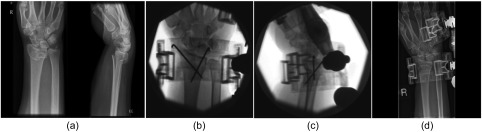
Radiographs and fluoroscopic images of a 35-year-old female patient with unstable intra-articular DRF (a) treated with non-invasive dynamic external fixator after closed reduction and percutaneous K-wire fixation (b and c). She had wires removed at three weeks post-op with continuation of non-invasive external fixator in dynamic mode (d) for further three weeks.
